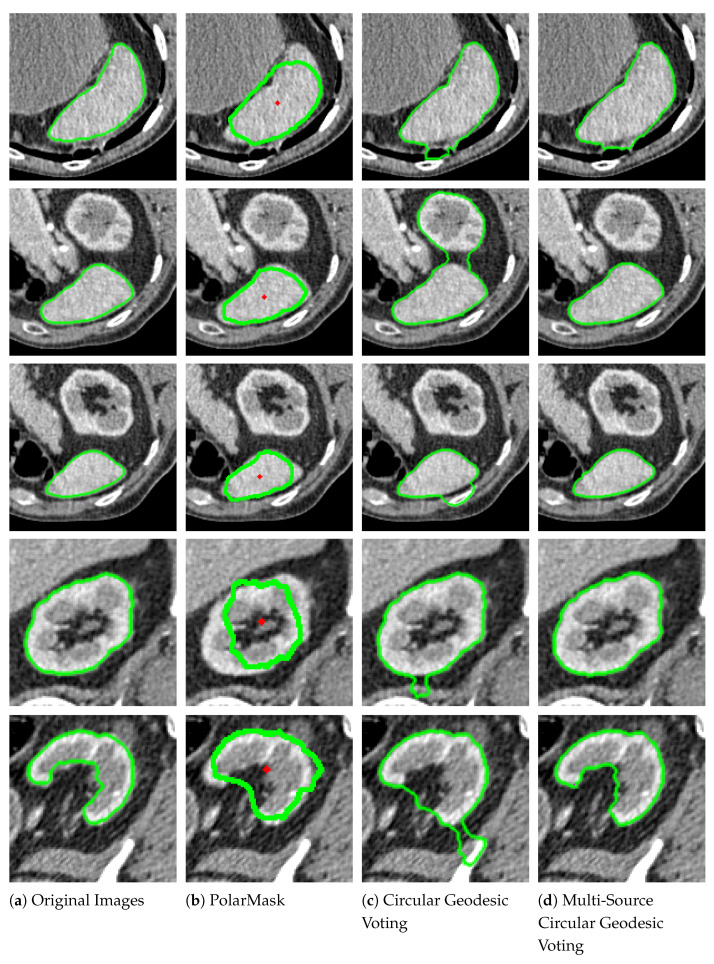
Qualitative comparison results on CT images. The green lines denote the segmentation contours. Column 1 displays the original images. Columns 2–4 show the segmented results produced by the PolarMask, circular geodesic voting, and multi-source circular geodesic voting methods, respectively.
